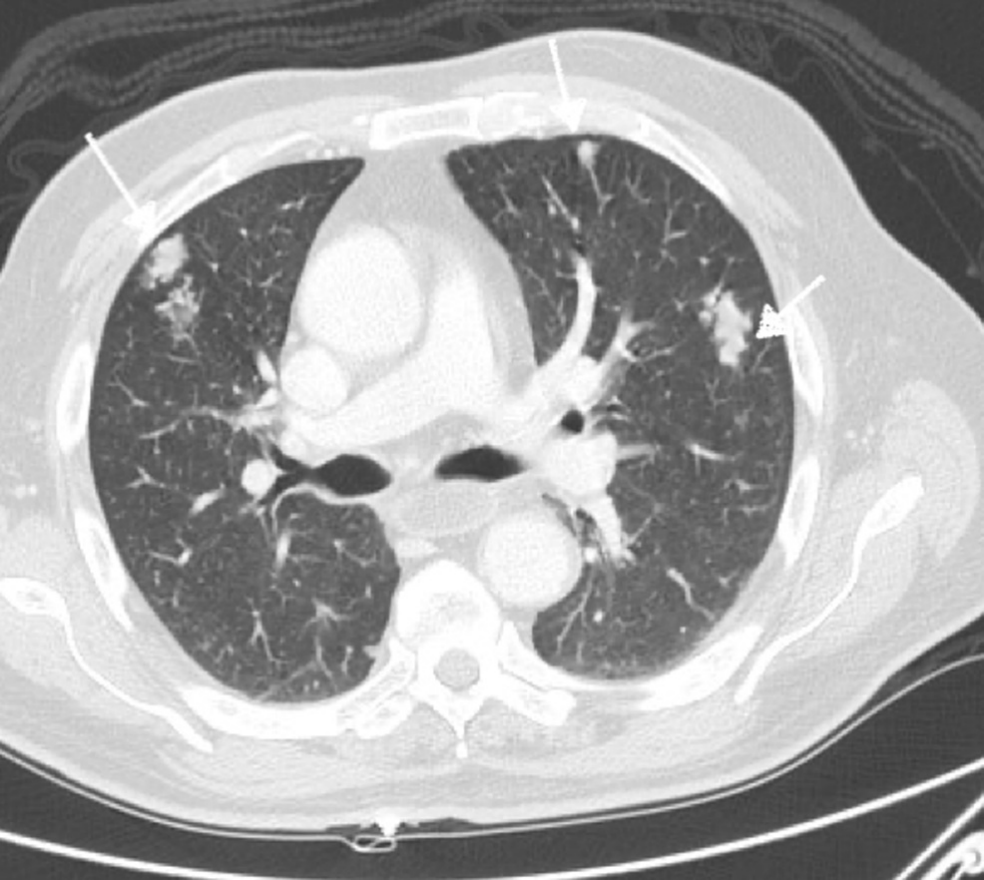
CT scan revealing bilateral pulmonary metastasis (Case 4). Arrows showing bilateral pulmonary metastatic lesions.
